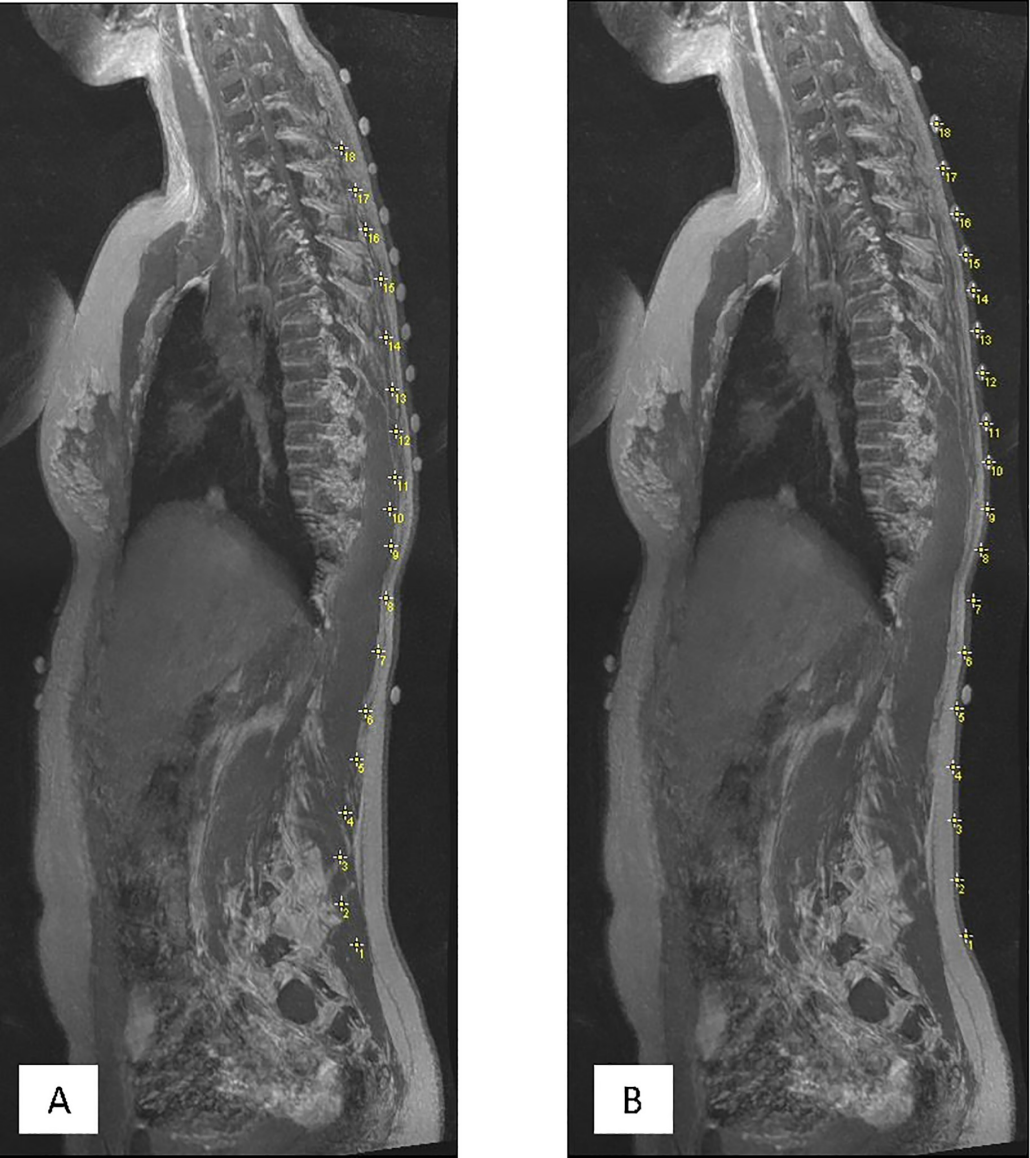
Sagittal plane maximum intensity projection. Sagittal plane projection (ImageJ) of the reformatted MRI data for Participant 9. (A) Yellow crosses demarcate the posterior margin of the spinous processes at each spinal level in the thoracolumbar spine; (B) Yellow crosses demarcate the centre of the fiducial marker attached to the skin surface over the thoracolumbar spine.
